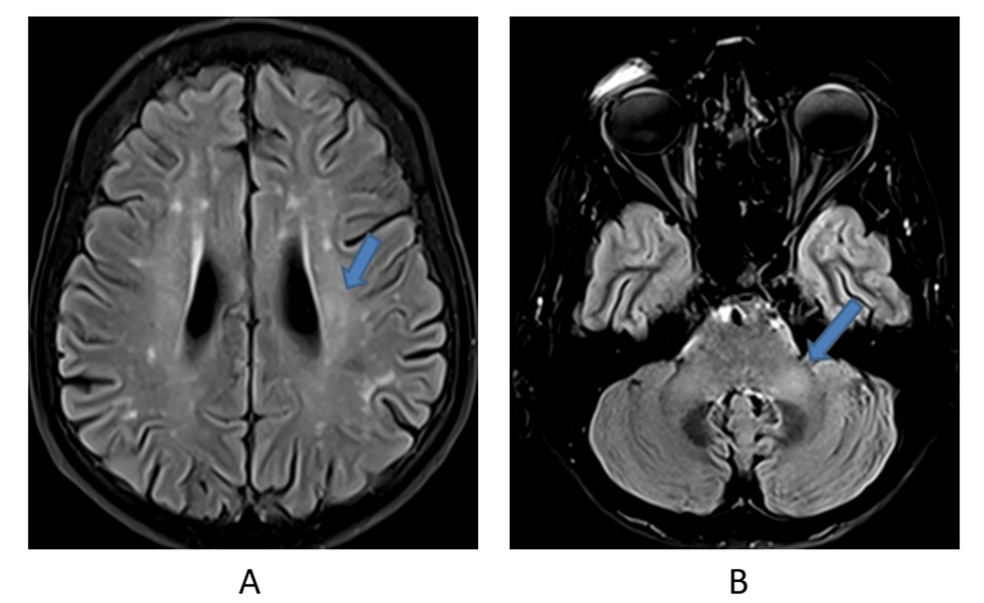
Image A is an axial FLAIR MRI of the brain showing a mild degree of white matter lesions, with faint periventricular hyperintensity (blue arrow). Image B is an Axial FLAIR MRI of the brain showing a mild degree of white matter lesions, with faint bilateral middle cerebellar peduncle hyperintensity (blue arrow). FLAIR: fluid-attenuated inversion recovery
